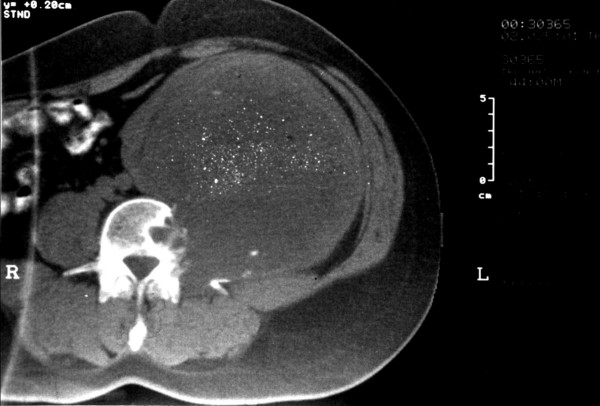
CT scan shows a 13.5 × 12 cm retroperitoneal mass eroding the left side of the 4^th ^lumbar vertebrae.
